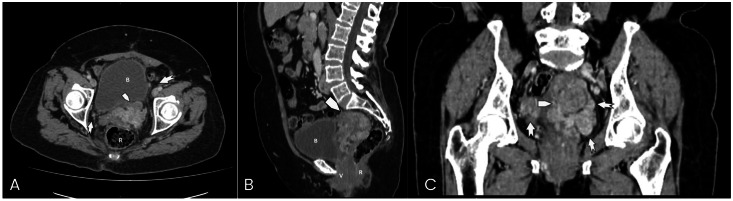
Uterine leiomyoma in a 74-year-old female patient. Contrast-enhanced venous phase abdominal CT. The images demonstrate a multilobulated pelvic mass (pentagon arrow) occupying the pelvic cavity, showing heterogeneous enhancement and loss of normal morphology due to multiple diffuse nodular lesions, some of them exophytic, with post-contrast enhancement. Adjacent pelvic lymph nodes (dentate arrow) with preserved morphology are also seen, all measuring less than 15 mm. A: axial view; B: sagittal view; C: coronal view. R: rectum; B: bladder; V: vagina.
